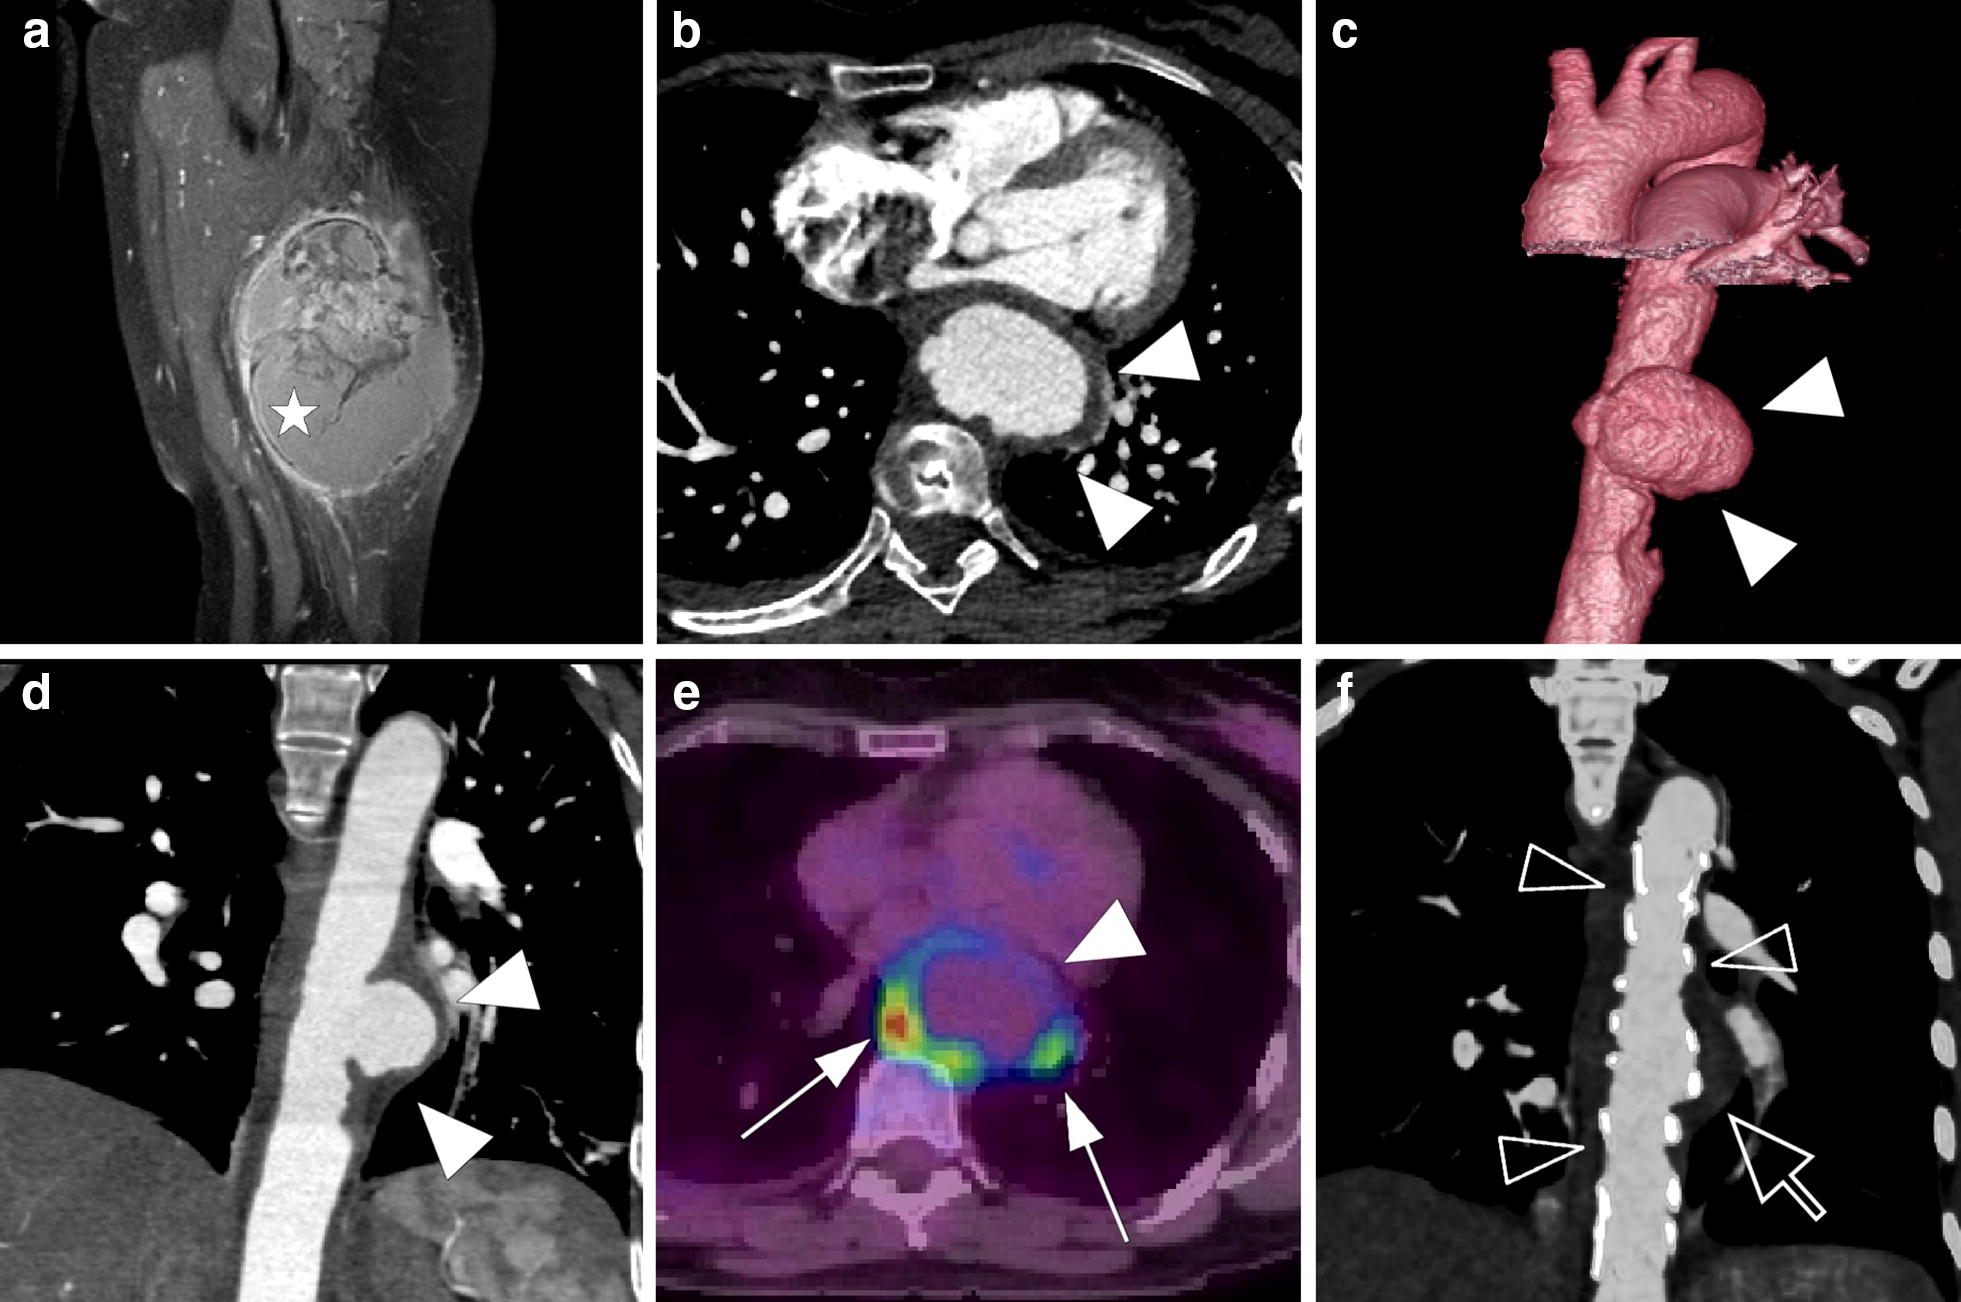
a Coronal gadolinium-enhanced T1-weighted image with fat saturation shows a posterolateral muscular mass of the left thigh with both necrotic and enhanced portions (white star); Saccular aneurysm of the descendant thoracic aorta (white head arrows): arterial phase CT scan of the aneurysm with axial view (b), coronal reconstruction (c) and 3D reconstruction (d). e PET-CT image fusion shows FDG uptake (white arrows) around the aneurysm. f Coronal maximum intensity projection (MIP) reconstruction of postoperative CT shows the endoprosthesis (transparent head arrows) and the excluded aneurysmal cavity (transparent arrow)
